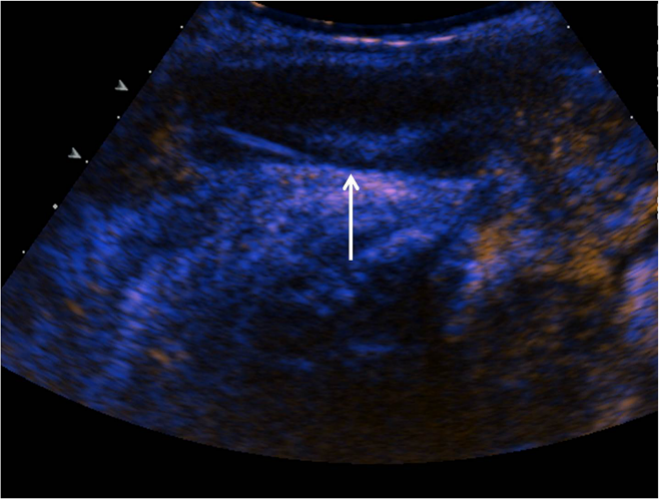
Contrast-enhanced ultrasound shows lack of perfusion and confirms the diagnosis of PSTCC (arrow).
